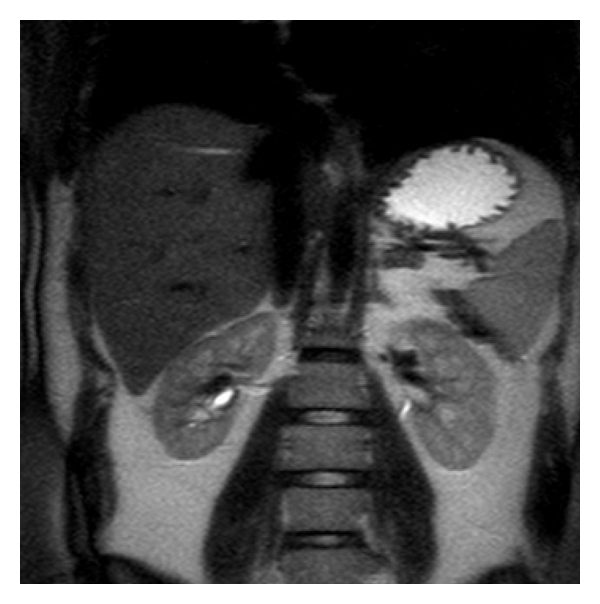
MRI of the right kidney, 6 months after the operation.
